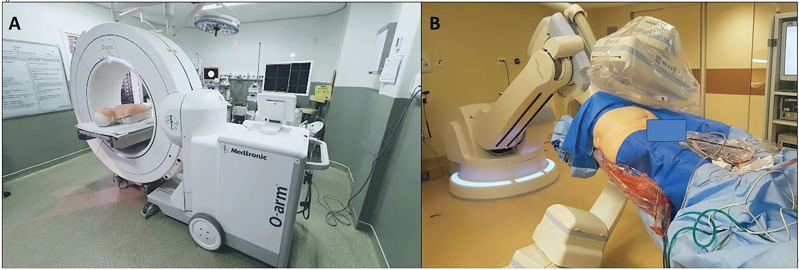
Demonstration of two models of cone beam intraoperative CT scans. 7-A – O-arm® imaging system (Medtronic Inc., Littleton MA), inside an operating room. 7-B – Uro Dyna CT® (Siemens Healthcare Solutions, Erlangen, Germany), showing its use at the end of a PCNL.
